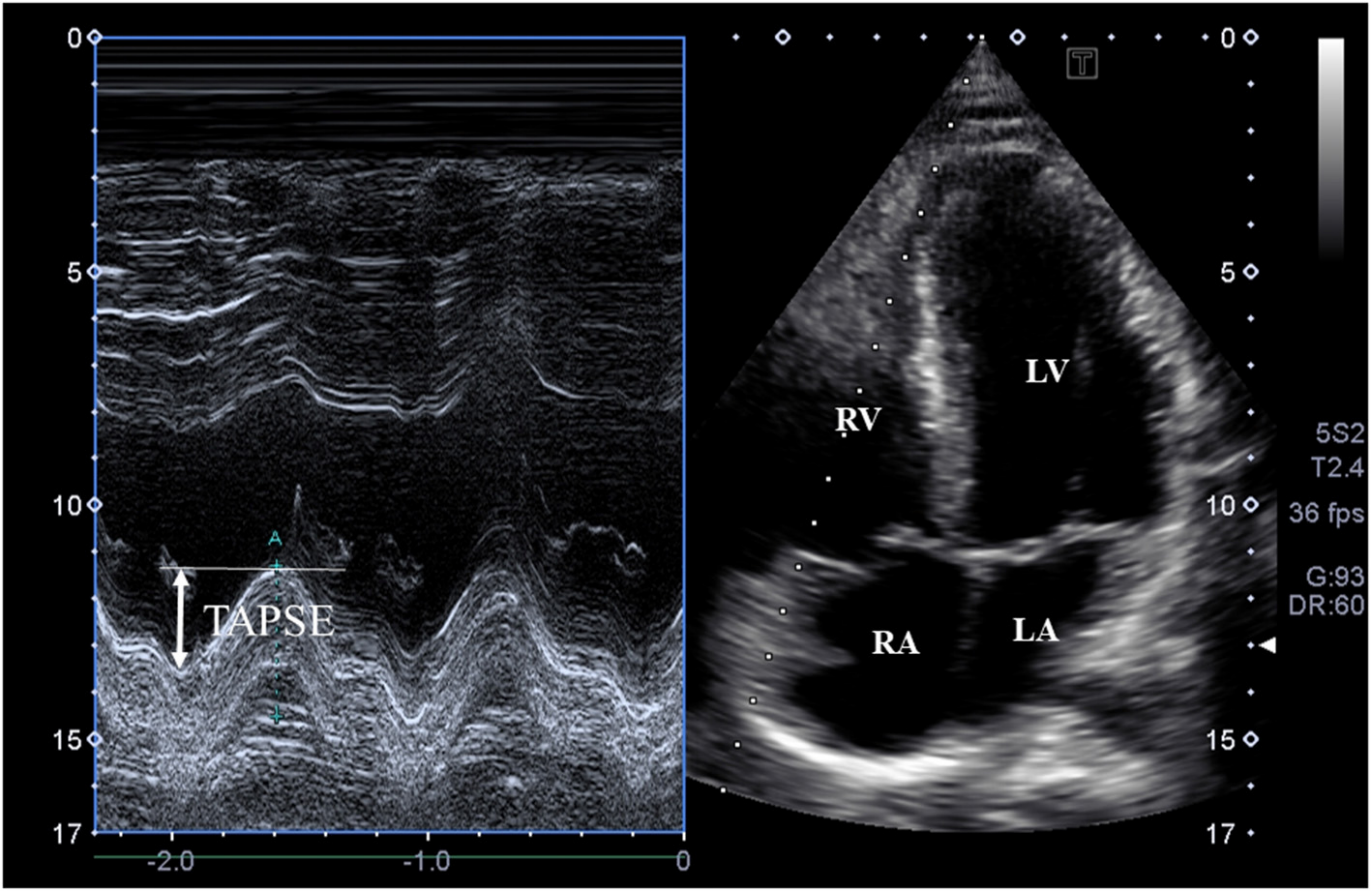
M-mode echocardiography-derived assessment of tricuspid annular plane systolic excursion (TAPSE) on apical four-chamber view.
